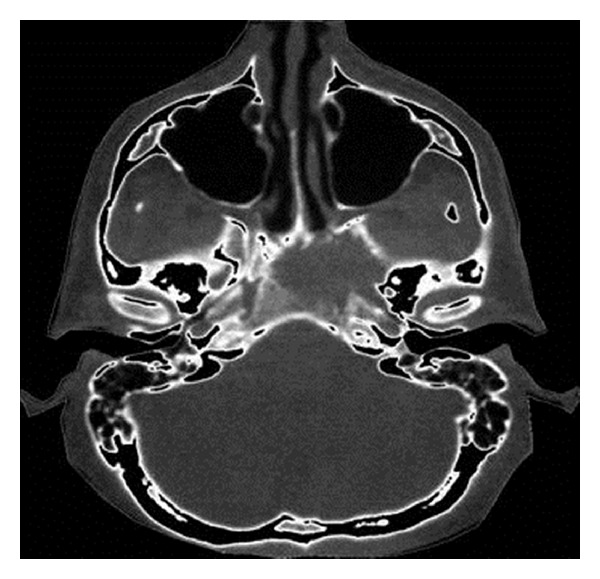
Cranial CT before radiotherapy.
